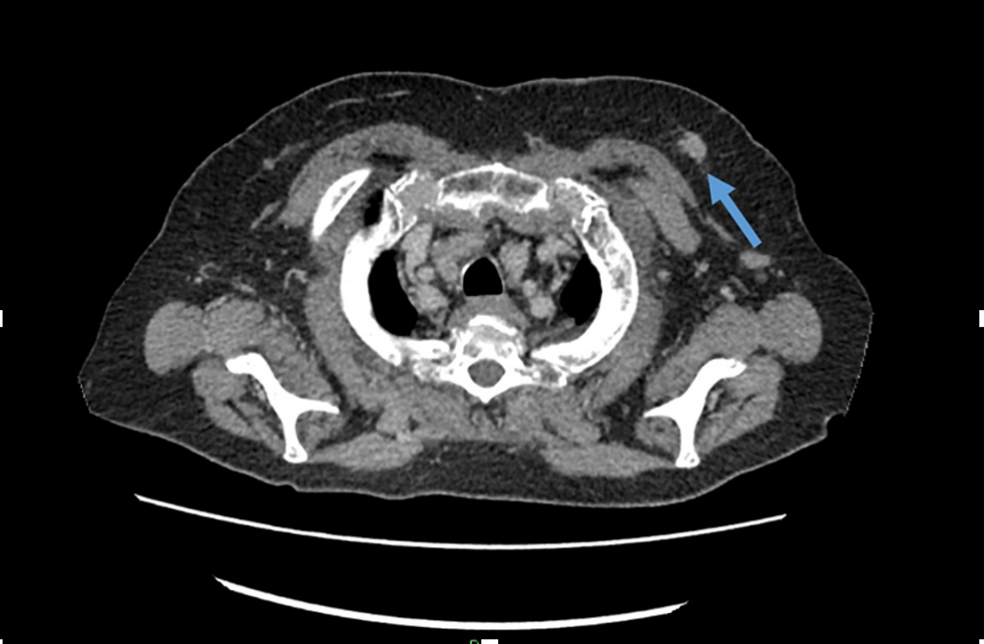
CT scan showing left upper quadrant breast lesion (arrow)
